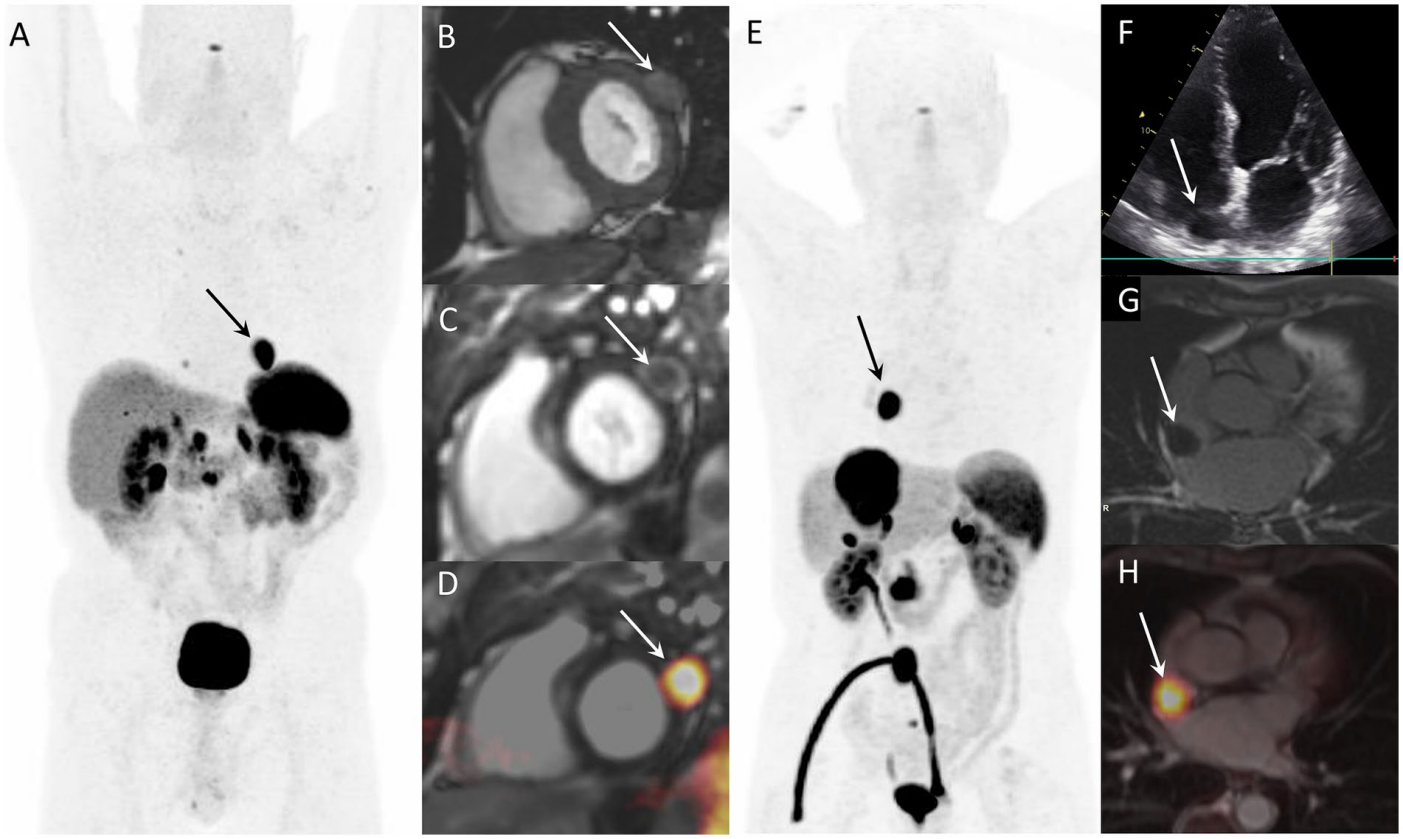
Examples of CNTM detected by SSTR-PET/CT in two NEN patients. Case 1: Myocardial metastasis of left ventricular latero-basal wall (arrows) detected by SSTR-PET/CT (A: anterior MIP) in a 74-year-old patient with G2 pancreatic NEN. The CNTM appeared at cardiac magnetic resonance (CMR) as a 22-mm nodule with intermediate intensity on T1-weighted images (B, short axis), with peripheral contrast-enhancement on phase sensitive inversion recovery sequence (C, short axis). Fused SSTR-PET/CMR image is also showed (D, short axis). Case 2: 76-year-old patient with inter-atrial myocardial lesion (arrows) of metastatic G1 small intestinal NEN detected by SSTR-PET/CT (E: anterior MIP). Echocardiography (4-chamber view) showed hypoechoic mass on the right side of interatrial septum (F). The CNTM showed a slight peripheral enhancement on delayed post-contrast inversion recovery CMR sequences (G, short axis). Fused SSTR-PET/CMR image is also showed (H, axial slice).
